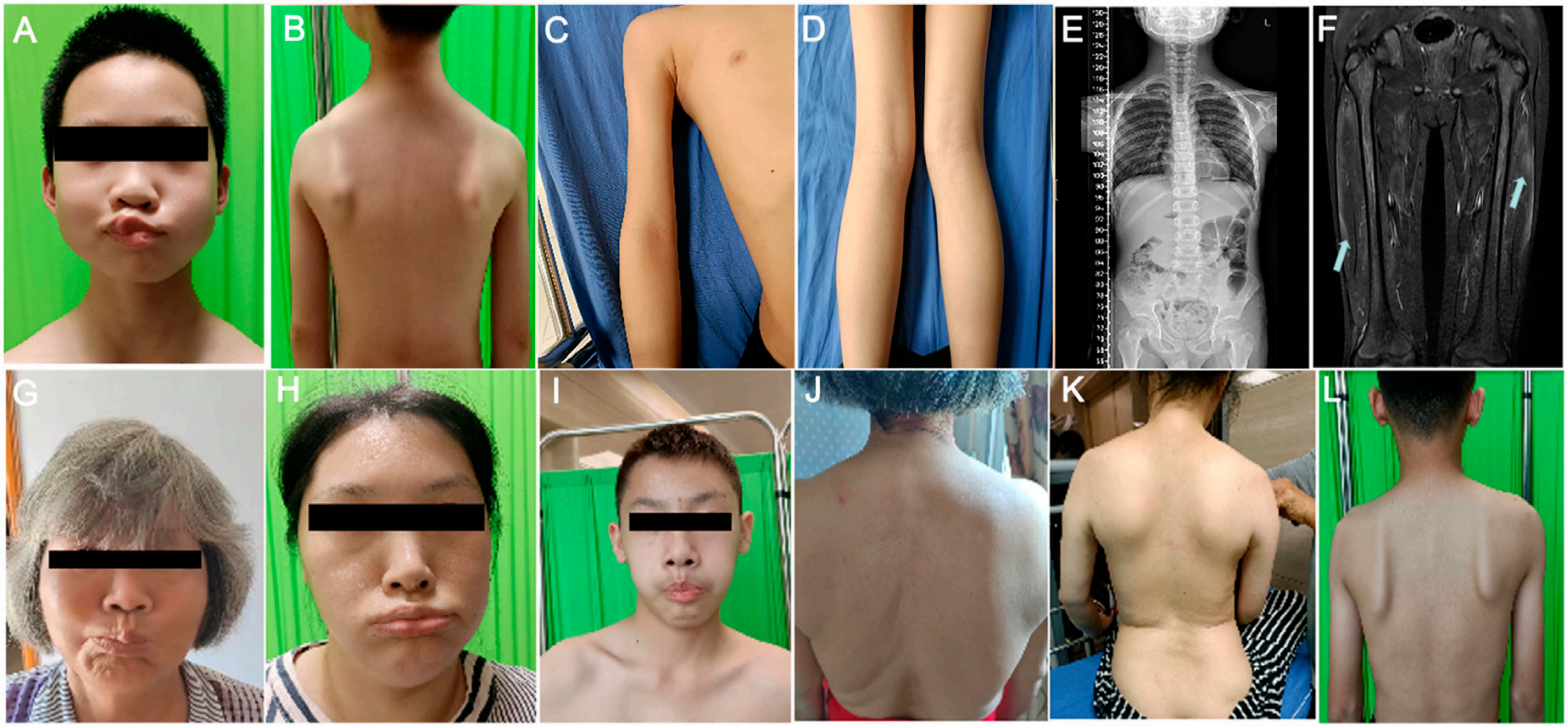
Clinical manifestations of the proband and his family members. (A) Facial features of the proband’s gills experiment. (B) Manifestations of the external shoulder blade of the proband. (C) Manifestations of proximal upper limb muscle atrophy in the proband. (D) The manifestation of proximal lower limb muscle atrophy combined with gastrocnemius pseudohypertrophy in the proband. (E) The entire spine of the proband shows X-ray results of mild scoliosis. (F) MRI of the proband’s bilateral lower limbs revealed widespread signal abnormalities in the thigh muscles, characterized by patchiness and high signal intensity in the T2WI fat suppression sequence. (G–I) The facial features of the proband’s maternal grandmother (G), proband’s mother (H), and proband’s cousin’s (I) bulging cheeks. (J–L) External shoulder blade phenotypes of proband’s maternal grandmother (J), proband’s mother (K), and proband’s cousin (L).
